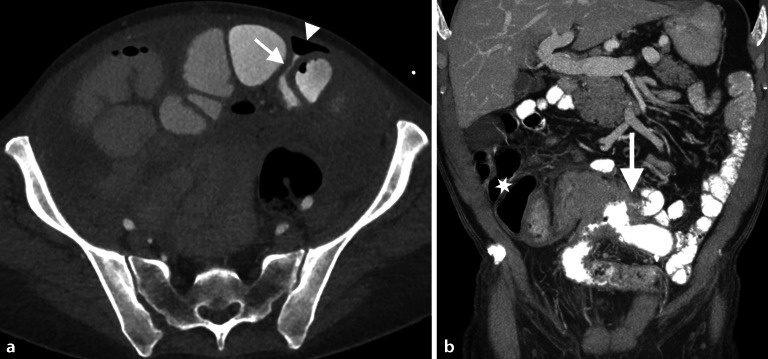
Zwei unterschiedliche Patienten mit positivem oralen Kontrastmittel. a Patientin mit peritoneal metastasiertem Appendixkarzinom nach hypertherm intraperitonealer Chemotherapie (*HIPEC*): Aufgrund steigender Entzündungsparameter und abdominaler Schmerzen erfolgte eine Computertomographie (CT), die ein kleines Dünndarmleak (*Pfeil*) mit begleitender extraluminaler Gasansammlung im ventralen Abdomen zeigt. b Patient nach Ileosigmoidostomie bei ausgedehntem Morbus Crohn und Bauchschmerzen sowie progredienter Diarrhö: Das koronale CT-Bild zeigt eine ausgedehnte Raumforderung im Bereich der Anastomose (verifiziert als Lymphom) und eine Fistelbildung (*Pfeil*); zu beachten ist das fehlende intraluminale Kontrastmittel vor der Anastomose durch die fistelbedingte Umgehung (*Stern*)
